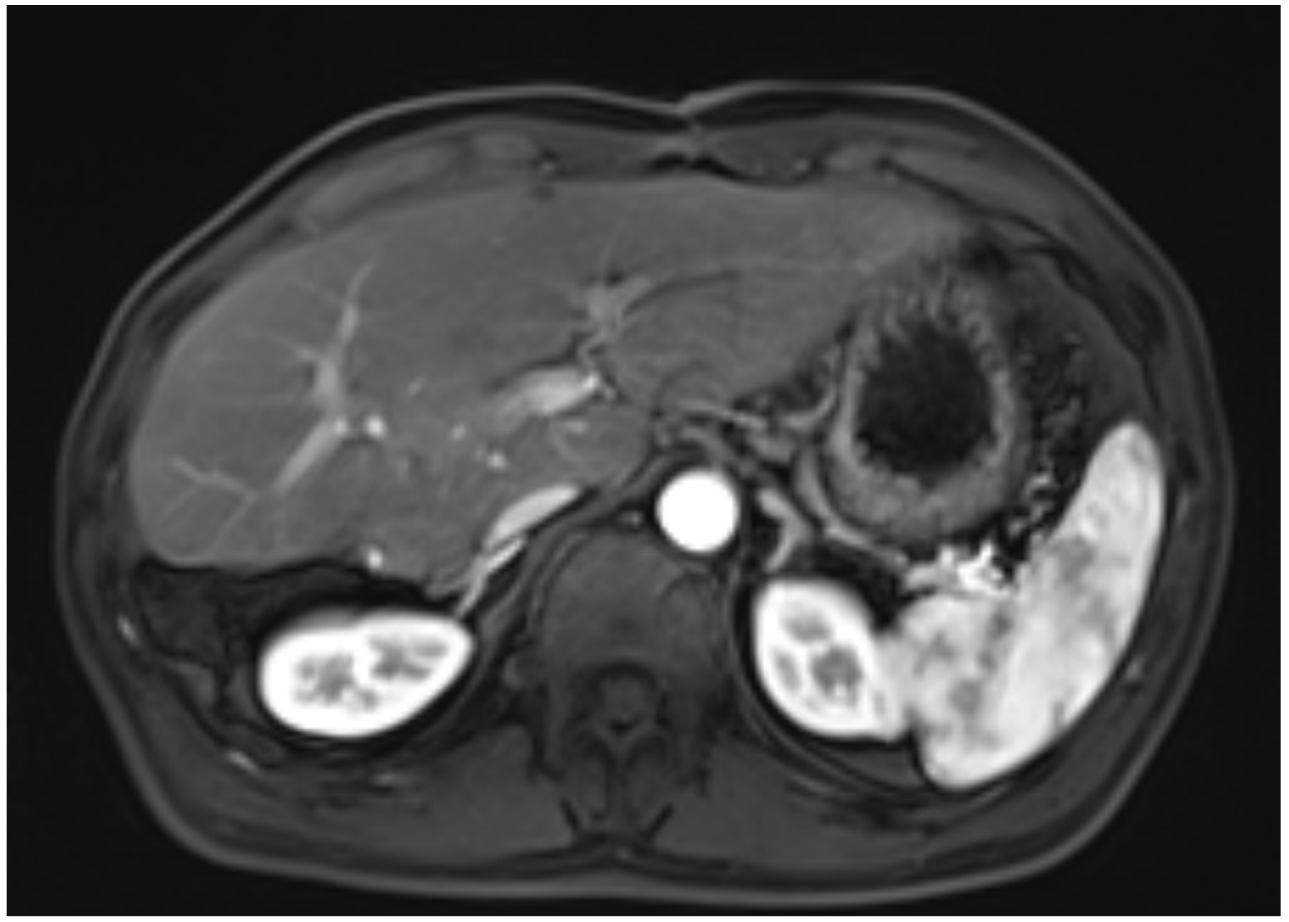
No recurrence was found on MRI 6 months after surgery.
